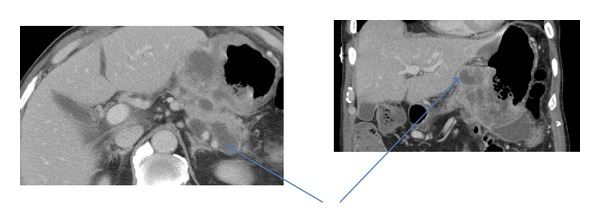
Computer tomography (CT) of the abdomen and pelvis showed a large heterogeneous mass involving the posterior gastric wall with diffuse extension into the gastric cardia. Two irregular hypodensities measuring 1.1 cm and 1.3 cm were identified in the inferior left hepatic lobe.
